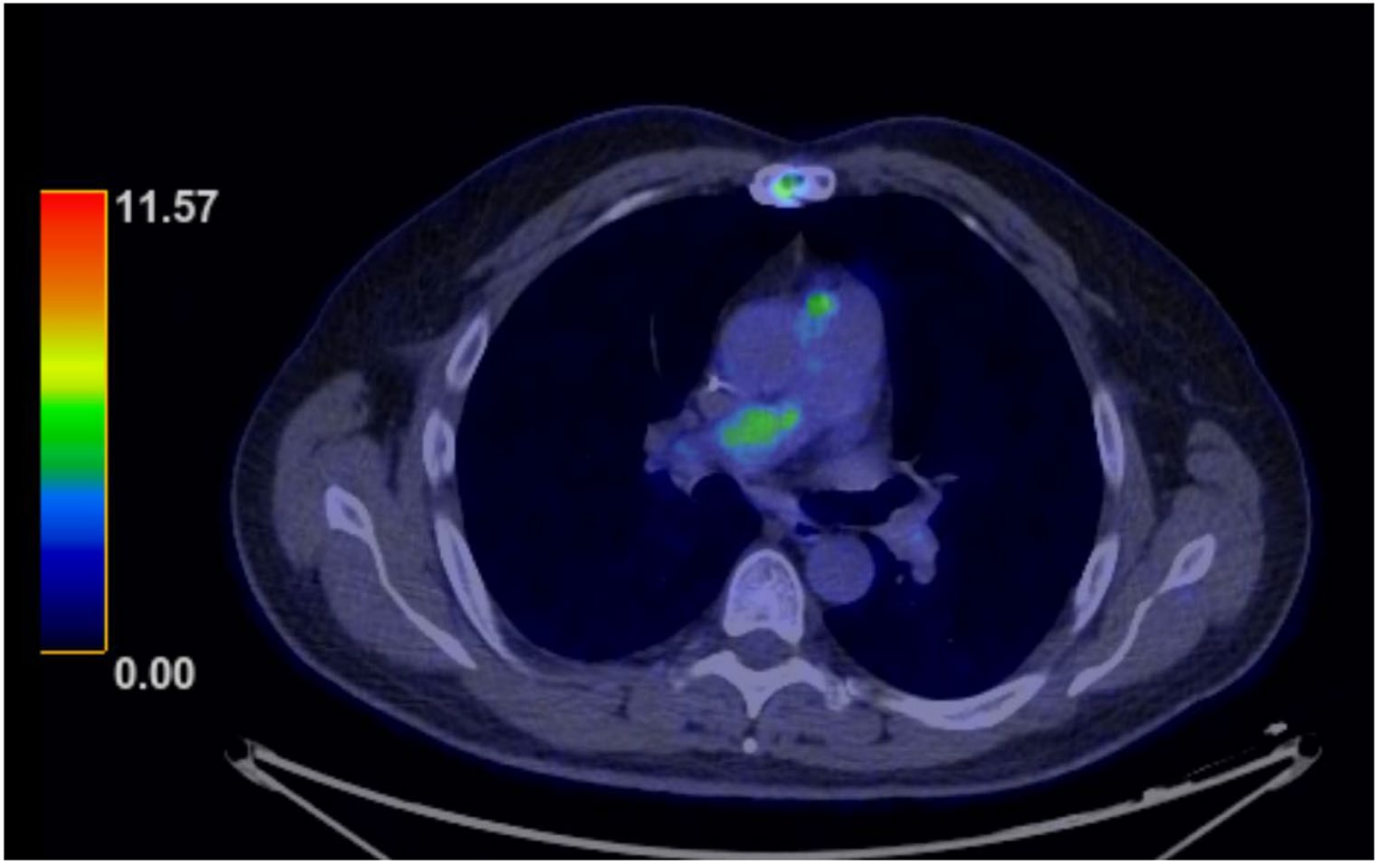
PET-CT showing an axial view of the recurrent, metabolically active mass in the pulmonary trunk
